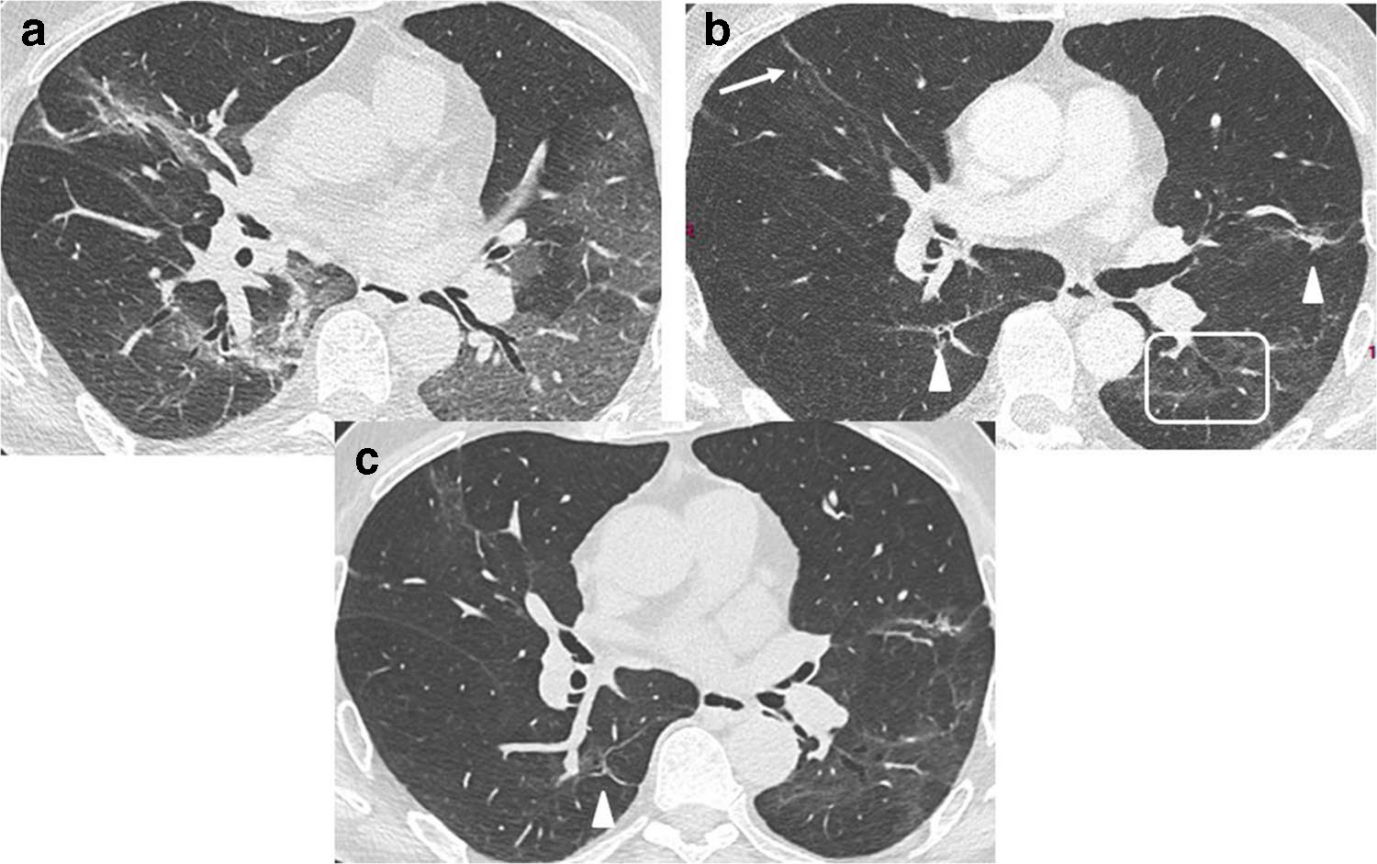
CT features at baseline and 4-month and 1-year follow-up, in a 65-year-old man who developed severe COVID-19 pneumonia (intubation, 27 days in ICU). a Baseline CT. b 4-month CT follow-up. Ground glass opacities (GGOs) have largely resolved, except in the upper segment of the left lower lobe. Bronchial dilatation (square) is demonstrated within the residual GGO area. Linear consolidation (arrow head) and parenchymal band (arrow) are also denoted. c 1-year follow-up. CT features are unchanged. There are no honeycombing and no peripheral reticulations
